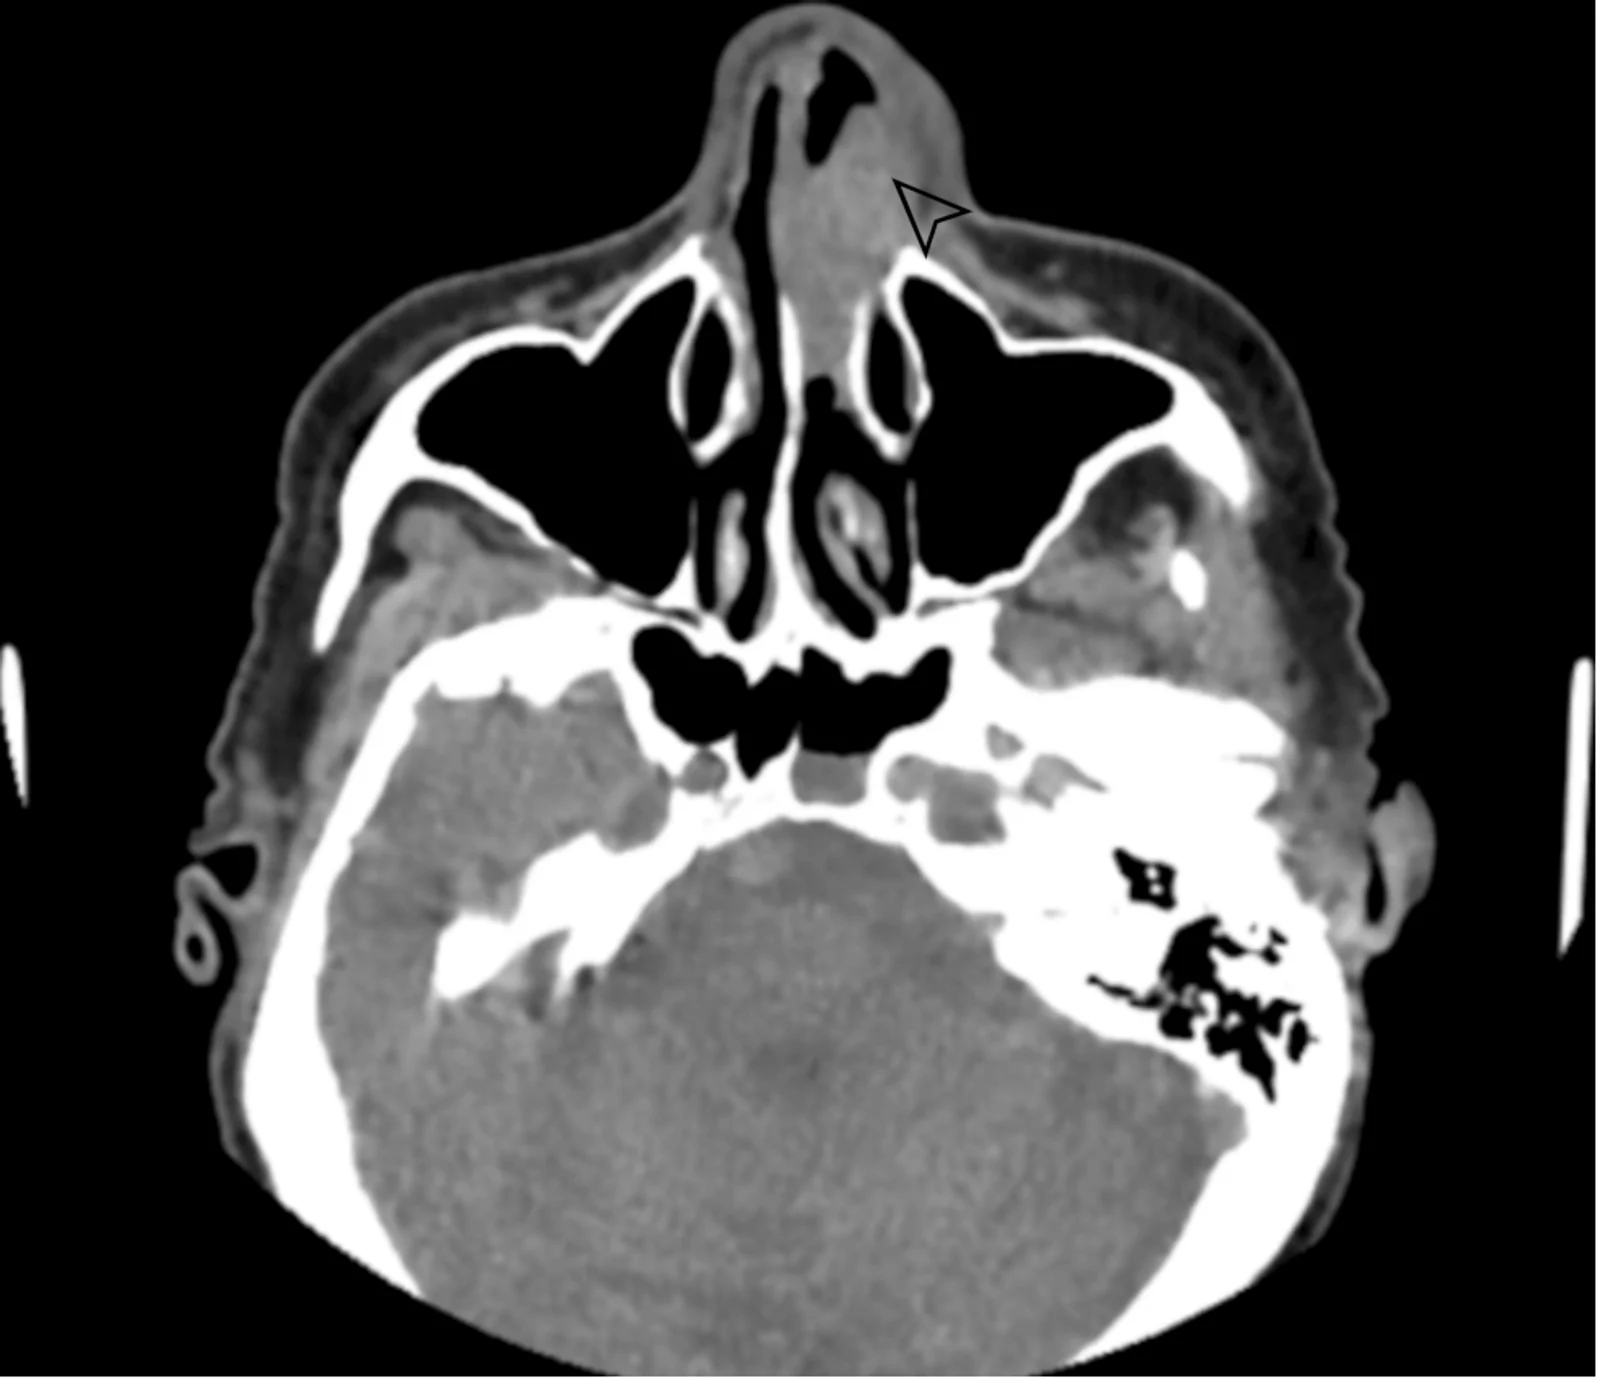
CT of the paranasal sinuses and nasal cavities (axial view) Axial contrast-enhanced CT image showing the same left nasal cavity mass (arrowhead), arising from the inferior turbinate and occupying the nasal cavity. No evidence of adjacent bone destruction or extension to surrounding structures was observed.
